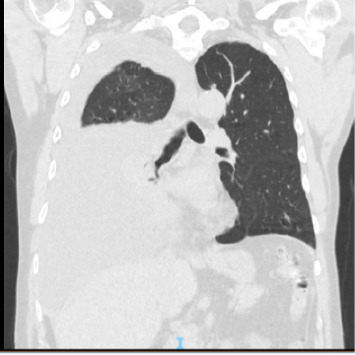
Chest CT scan showing severe unilateral pleural effusion.
